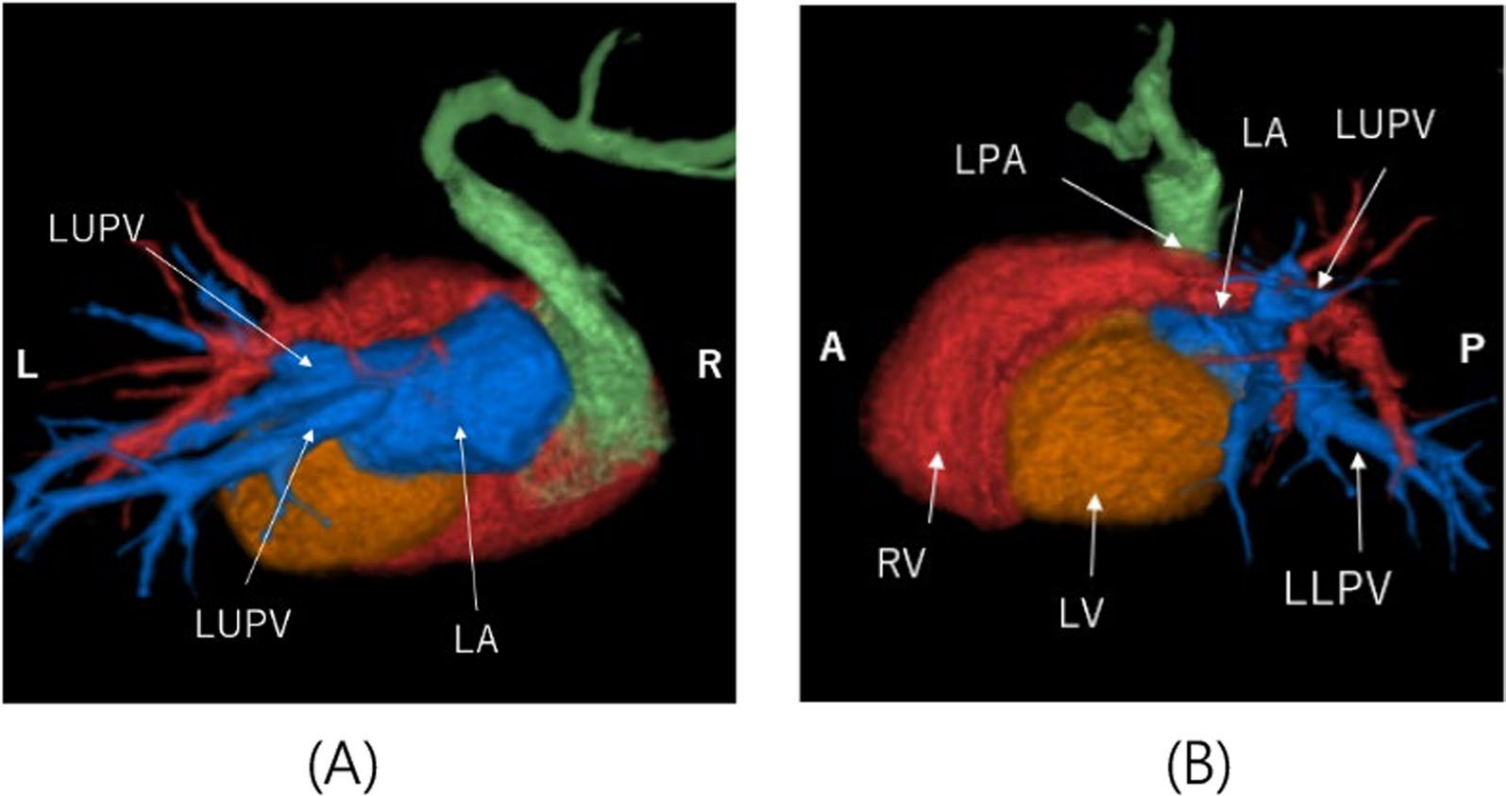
Postoperative 3-dimensional computed tomography. A, B The left pulmonary veins connected to the left atrium (posterior to anterior view and left side to right side view). LUPV, left pulmonary vein; LLPV, left lower pulmonary vein; LPA, left pulmonary artery; VV, vertical vein; InnV, innominate vein; RV, right ventricle; LV, left ventricle; LA, left atrium; LPA, left pulmonary artery
